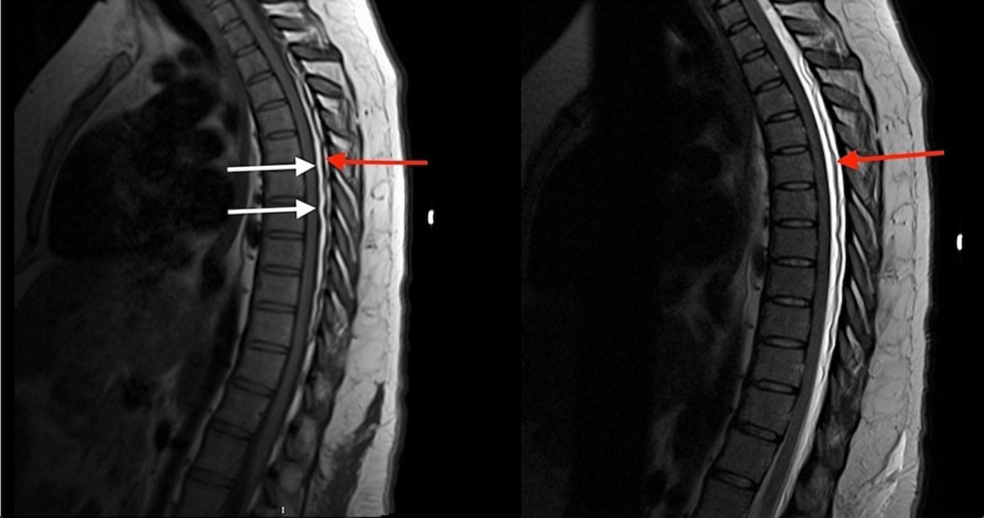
Sagittal T1-weighted MRI (left) and T2-weighted MRI (right) show a thick proliferation of epidural fat (white arrows) compressing and displacing the dural sac toward the anterior and epidural vein engorgement (red arrows) MRI: magnetic resonance imaging
